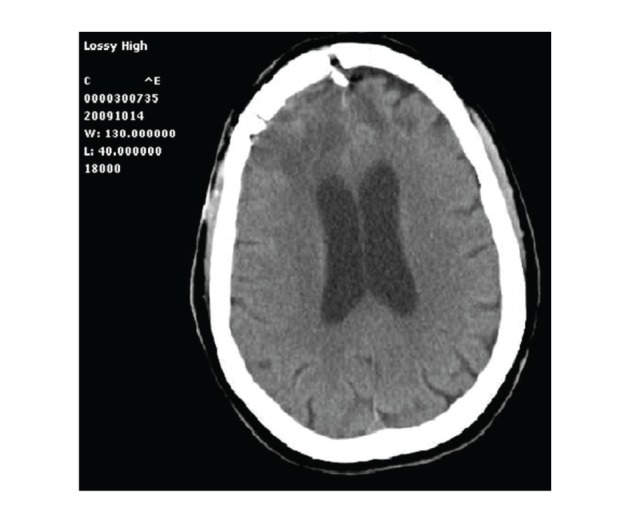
Axial CT scan few days after granuloma excision: note the dramatic but incomplete reduction of brain edema.
